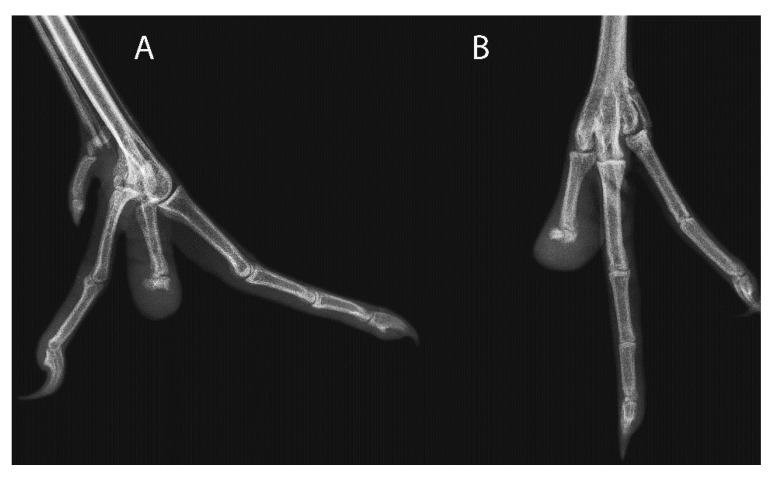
(A) Lateral and (B) dorsoplantar radiographs of the left foot of Case 1, a 13-year-old female black-necked crane (*Grus nigricollis*) with cellulitis and probable osteomyelitis of the fourth digit of the left foot at time of presentation.
